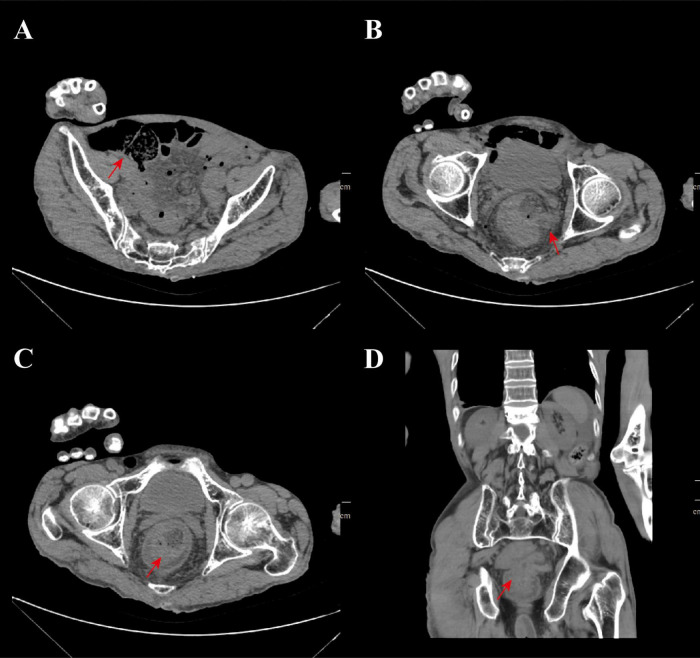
CT imaging findings. (A) The CT imaging manifestations of small intestine dilation and inflation, the red arrow indicates the dilation of the small intestine; (B) imaging manifestations of small intestine prolapse after rectal rupture and the red arrow indicates the site of rectal rupture; (C) the imaging shows that there is intussusception and rectal prolapse and the red arrow indicates the lesion site; (D) the coronal plane imaging manifestations of the patient, the red arrow indicates the location of recurrent rectal prolapse and intussusception.
